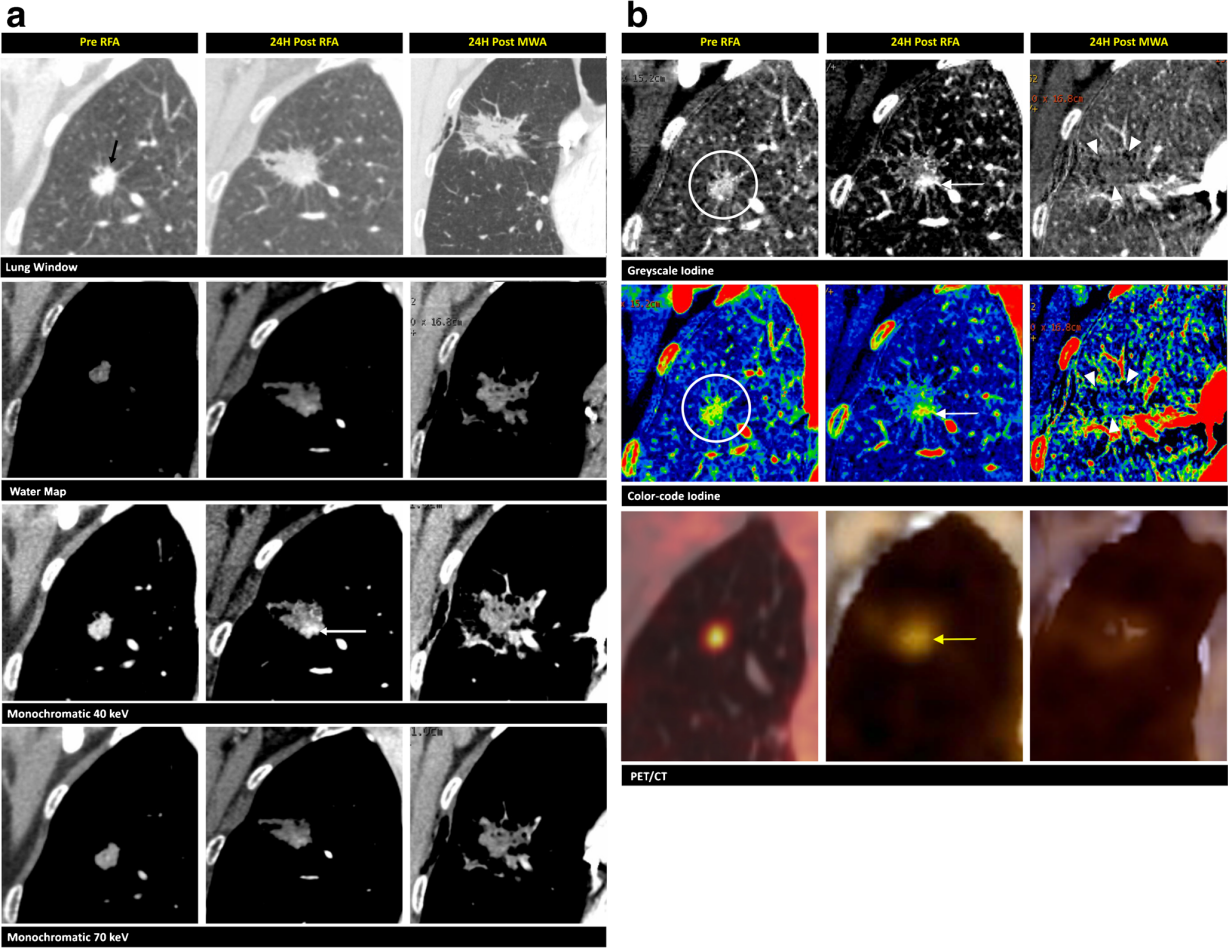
Pulmonary metastases. A focal lung nodule is found in a 65-year-old female diagnosed with ovarian carcinoma. A biopsy reveals a non-small-cell carcinoma. A multidisciplinary team meeting leads to the choice for RF ablation treatment. Coronal reconstructions of the lesion in the right upper lobe before (first column), 24 h after the first RF ablation (second column), and 24 h after microwave ablation (third column) are demonstrated. a A focal nodule of 9 mm in the upper lobe is seen on the 70-keV lung window (*black arrow*) prior to the ablation. This nodule is “enhanced” on the synthesised monochromatic images compared to the water map image. This “increase in density” is better appreciated on the 40-keV rather than on the 70-keV images. Twenty-four hours after the first ablation, the spicular nodule appears enlarged on the lung windows. On the 40-keV image, a focal enhancement (*arrow*) is noted at the inferior border of the ablation zone, again more clearly visible compared to the 70-keV image. After re-ablation with microwave technique, we notice more important changes on the lung window than after the first ablation. b The iodine content of the hypermetabolic lesion before the RF ablation is most observable on the greyscale- and colour-coded iodine images (*circles*). The nodule is hypermetabolic on PET/CT. Twenty-four hours after the first ablation, the greyscale- and colour-coded iodine maps clearly show the focal area of iodine uptake (*white arrows*) on the inferior border. PET/CT confirms hypermetabolic remnants (*yellow arrow*). After re-ablation, the larger area of thermal damage has lost its internal focal iodine uptake, which is most appreciable on the iodine-coded images (*arrowheads*). Images after the re-ablation lack FDG uptake on PET/CT
